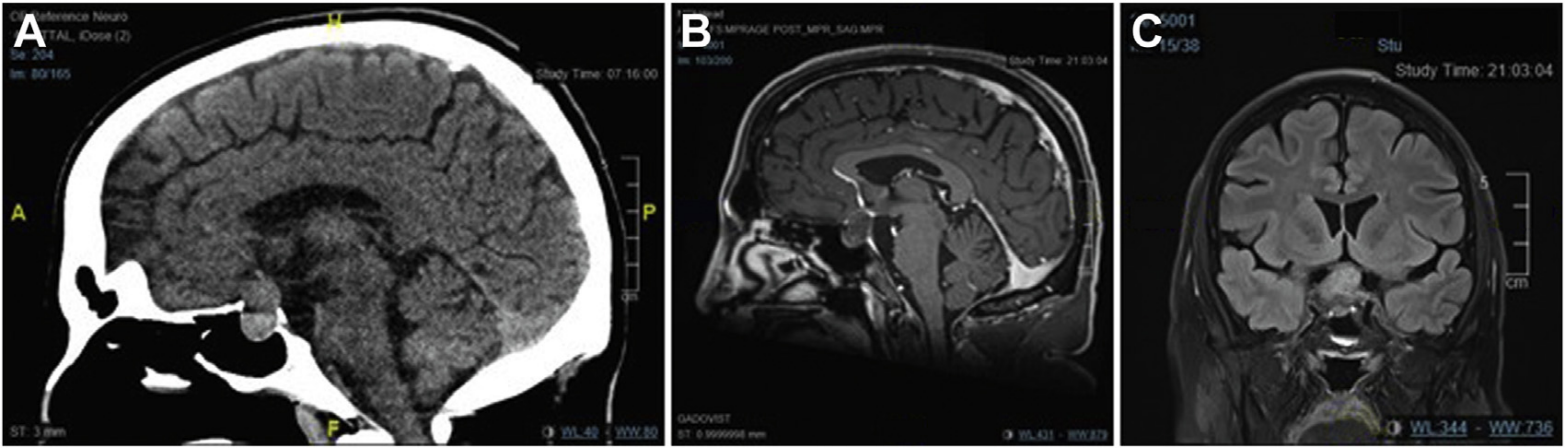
A*, Hyperattenuating 2.0 × 2.8 × 1.5-cm mass at the sella turcica on unenhanced computed tomography. Magnetic resonance imaging revealed a 1.9 × 3.2 × 2.4-cm heterogeneous mass on, (*B*) T1-weighted imaging and (*C*) T2-weighted imaging showing small hyperintense areas in the solid part of the sella mass with flattening of the optic chiasm and remodeling/dehiscence of the floor of the sella, extending into the right cavernous sinus with at least partial encasement of the internal carotid artery. *ALF* =; *FLP* =; *HPR* =; *HRA* =; *RFA* =.
